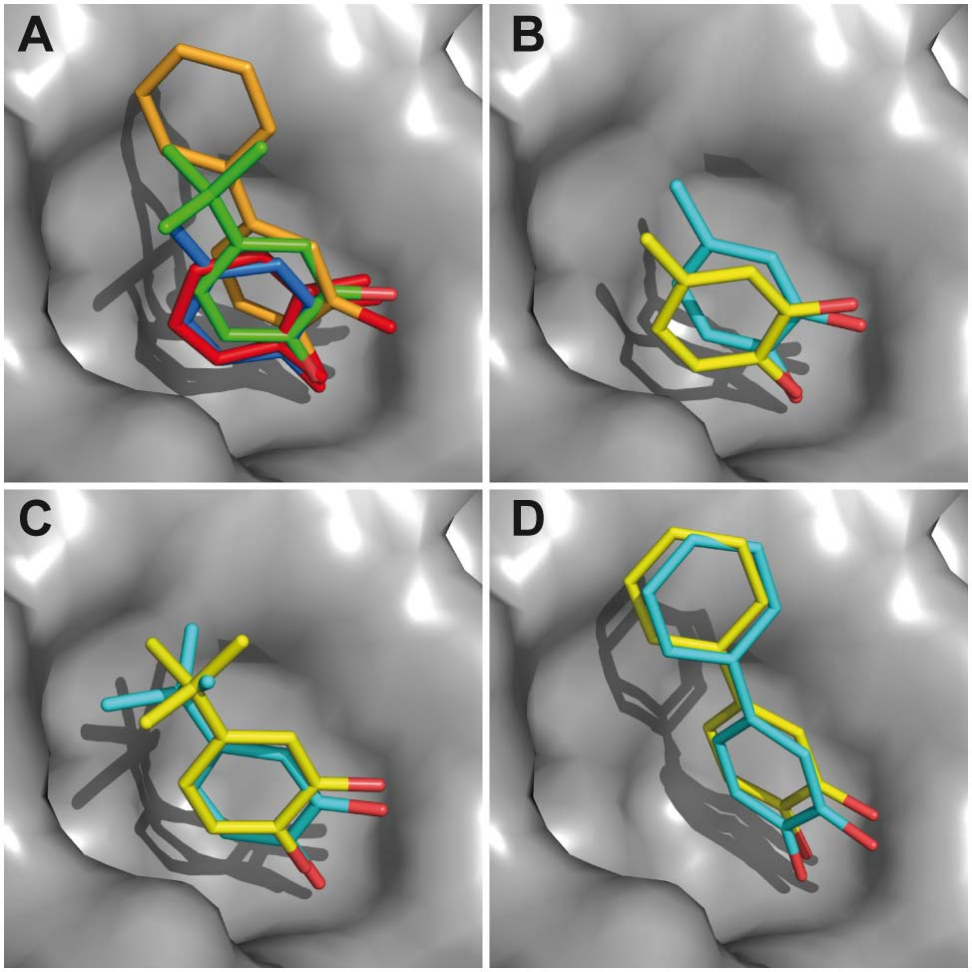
Comparison of NMR and X-ray protein-fragment structures. (A) Superposition of the X-ray structures of the complexes for fragments 1 (red), 2 (blue), 3 (green) and 4 (orange). (B) The NMR-derived binding modes (cyan) are compared to the X-Ray structures (yellow) for fragments 2 (B), 3 (C) and 4 (D). Fragment positions were extracted from the solved X-Ray structure and are displayed in the 3MNG protein structure (used for docking).
